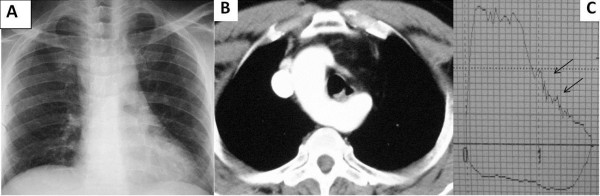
Thoracic MRI and spirometry images; right sided arcus aorta as seen on thoracic MRI (3A, 3B). Spirometry showed the “saw-tooth” sign (black arrows) and a plateau on flow-volume curves throughout exhalation and inhalation (3C).
